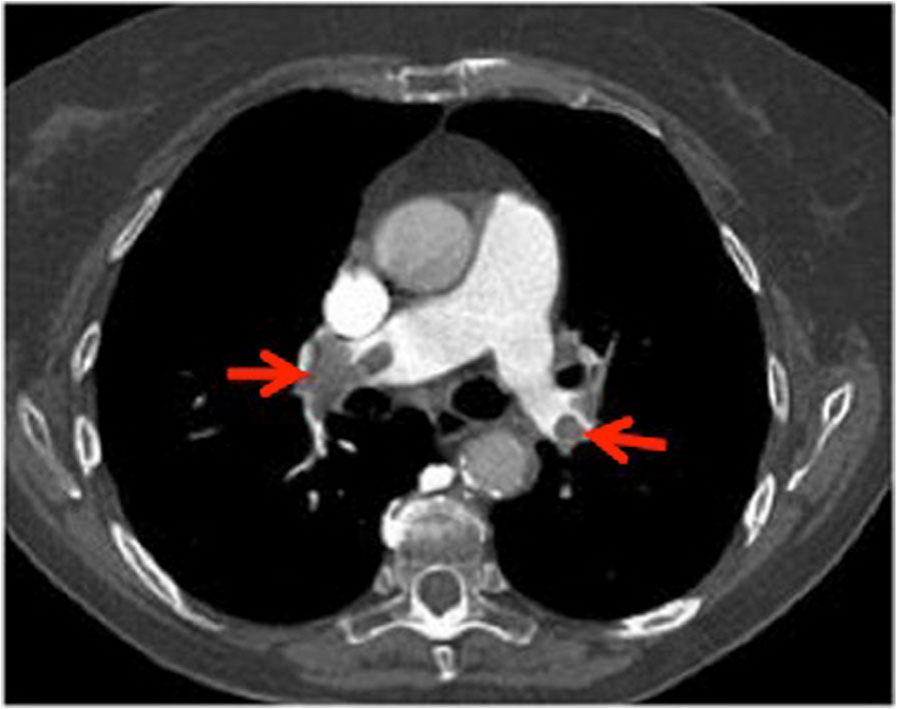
Contrast-enhanced computed tomography pulmonary embolism protocol. Massive bilateral thrombosis in the main pulmonary arteries (*arrows*)
